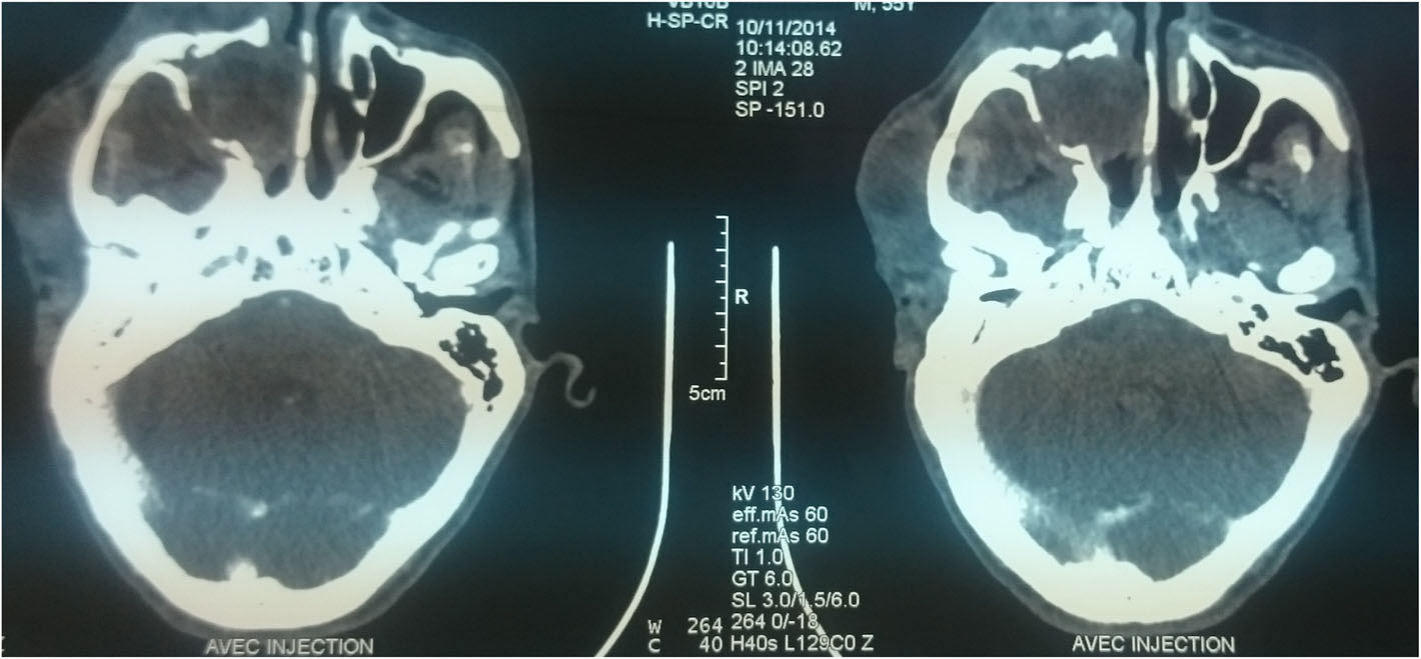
Computed tomography scan shows a mass in the right orbit
